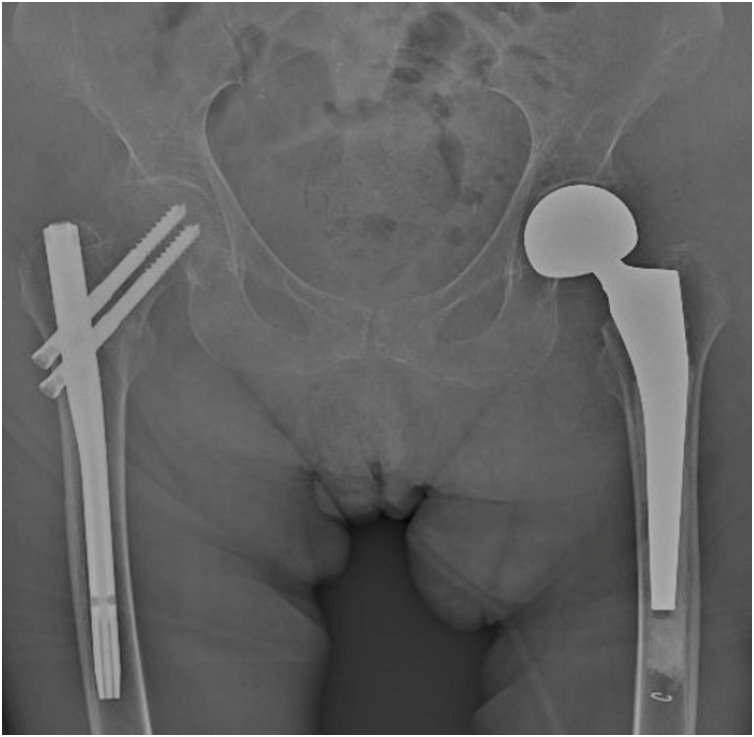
Postoperative radiographic examination of the hip in AP view of the same patient surgically treated by ORIF with an intramedullary nail on the right and by hemiarthroplasty on the left.
